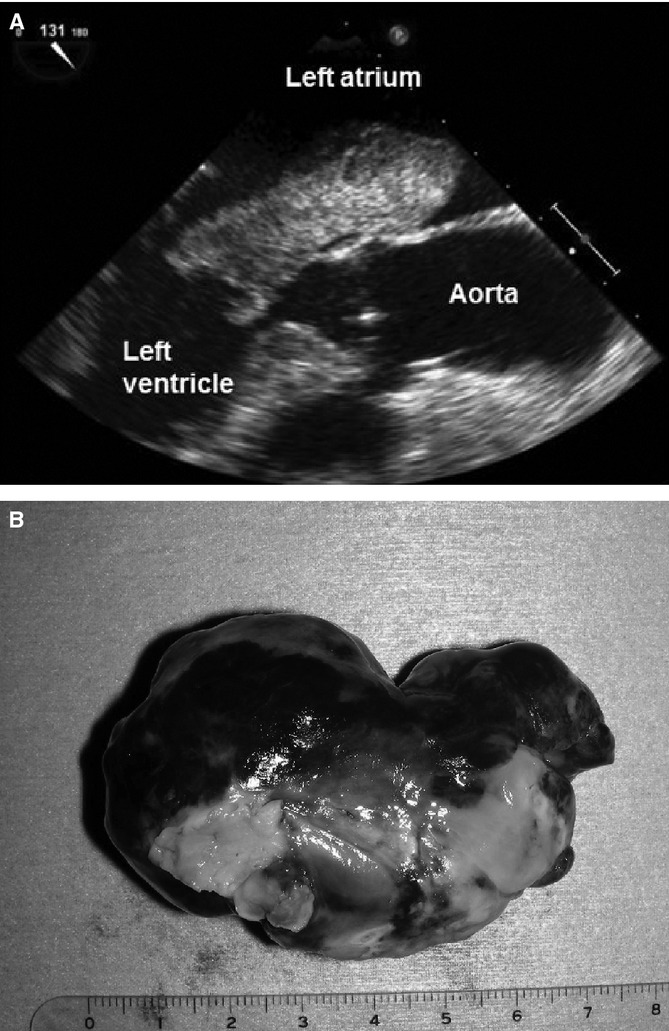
(A) Transesophageal echocardiogram showing an atrial mass prolapsing into the left ventricle. (B) The excised myxoma (3.5 × 4.5 × 7 cm).
